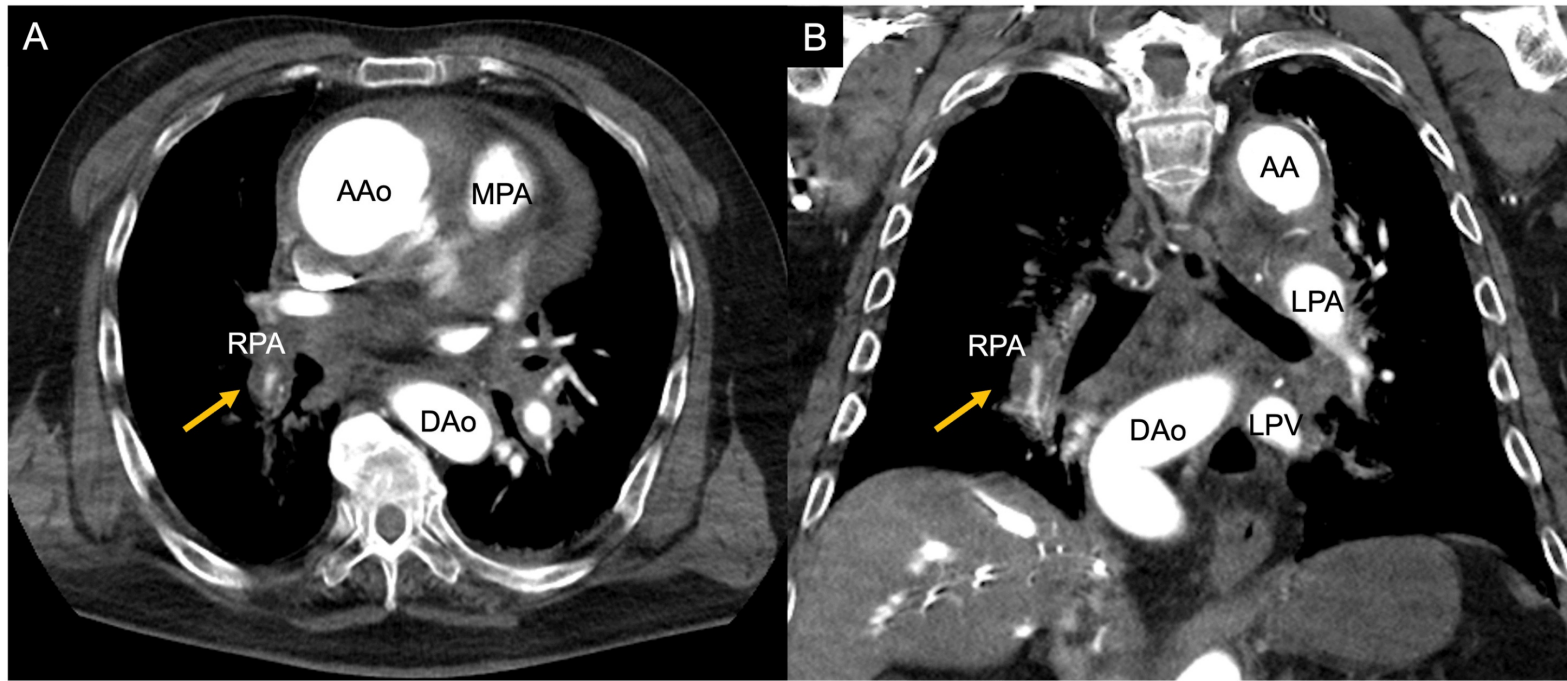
Contrast-enhanced computed tomography of a branch of the right lower lobe pulmonary artery On axial (A) and coronal (B) views, the arterial wall of the right lower lobe pulmonary artery branch is thickened, causing concentric compression of the lumen (yellow arrow). AA: aortic arch; AAo: ascending aorta; DAo: descending aorta; LA: left atrium; LV: left ventricle; RPA: right pulmonary artery
